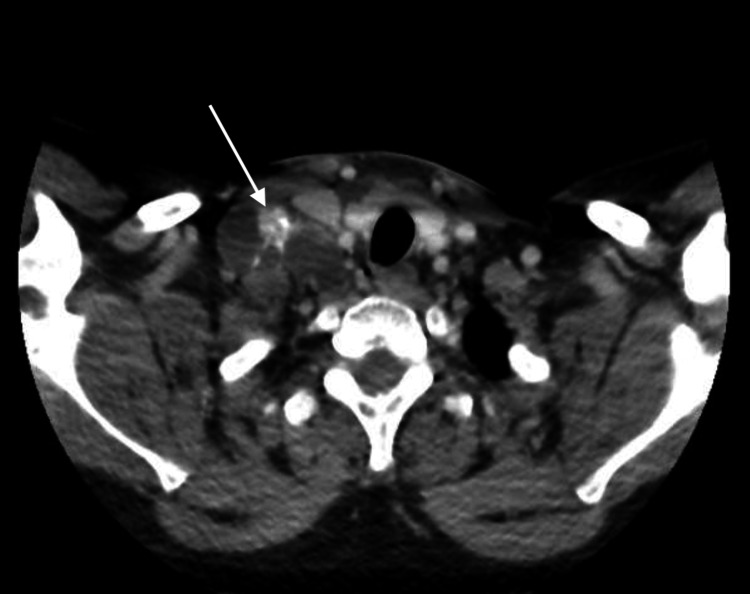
Contrast-enhanced CT neck Shows right cervical lymph node enlargement (white arrow). CT: Computed tomography.
